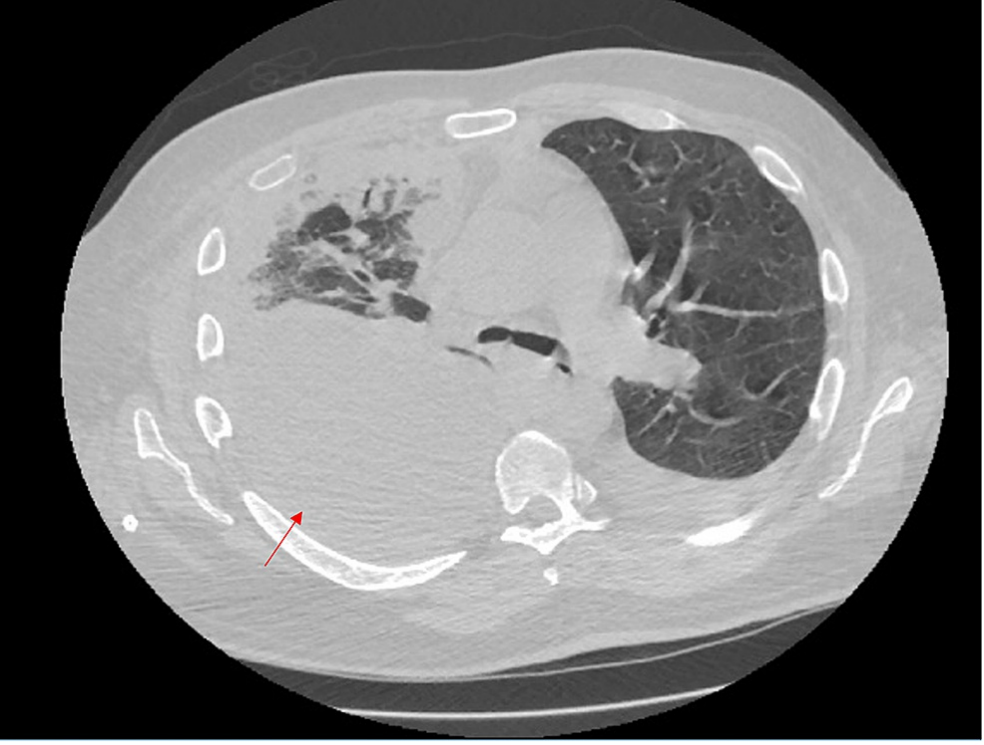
Computed tomographic scan of Chest showing loculated right-sided pleural effusion and left-sided pleural effusion
